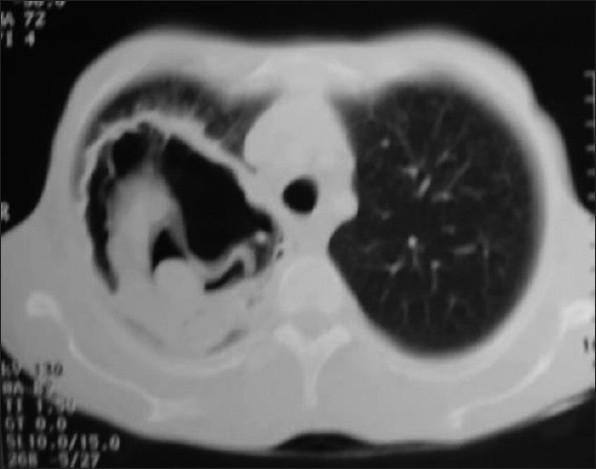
CT thorax showing cavity and its contents
